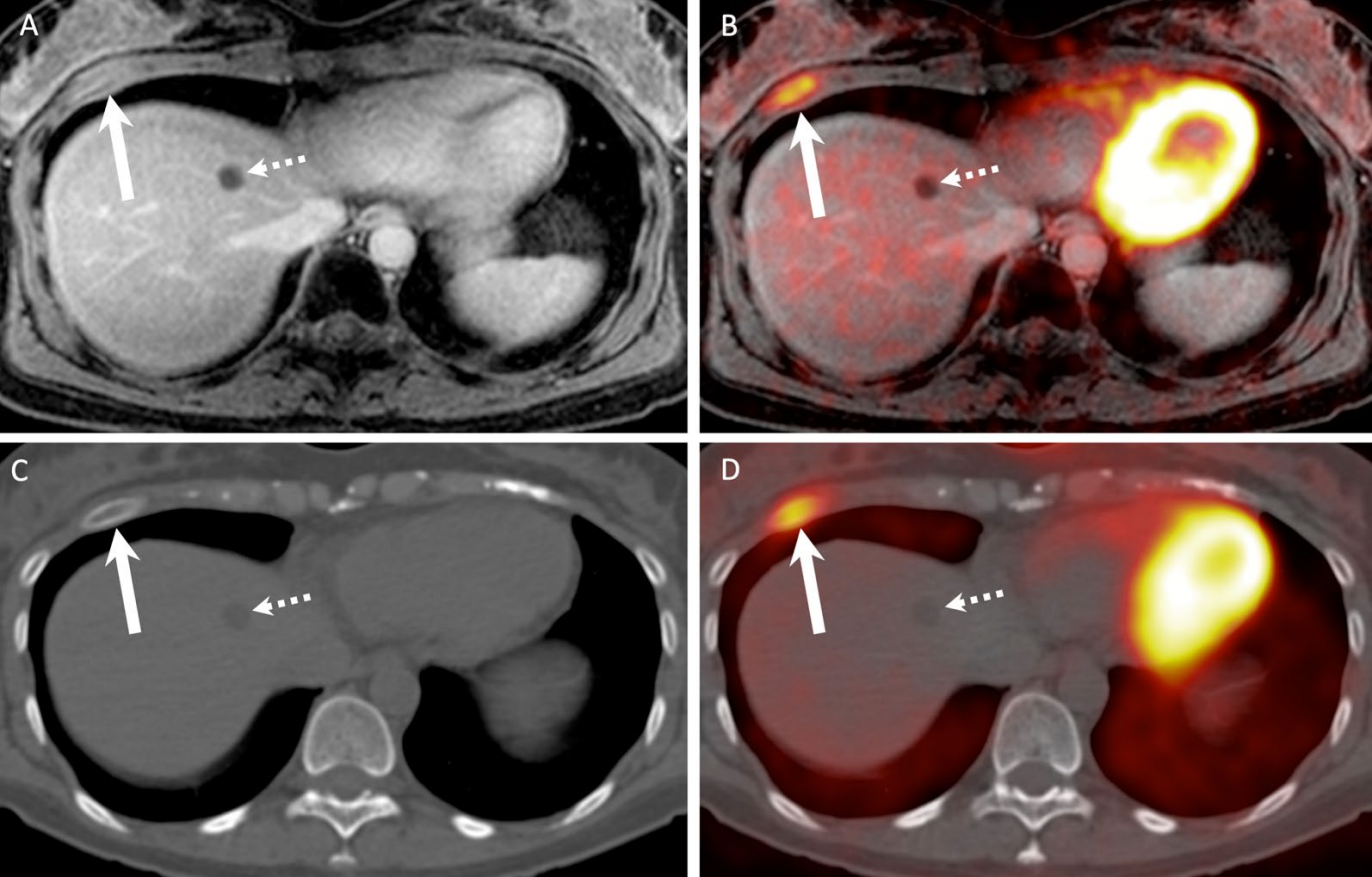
Rib metastasis detected on both PET/MRI (A, B) and PET/CT (C, D) in a 42-year-old female without loco-regional recurrence after radiochemotherapy for a SCC of the paranasal sinuses. The rib lesion (arrows) shows a combination of high focal [^18^F]FDG uptake (SUVmax on PET/MRI = 6.3 and SUVmax on PET/CT = 4.7) and an expansile aspect on MRI/CT (arrows). Note lesion enhancement on the contrast-enhanced fat-saturated MR image. The lesion was rated with a score of 5 on PET/MRI and with a score of 4 on PET/CT. Dashed arrows point at a liver cyst
